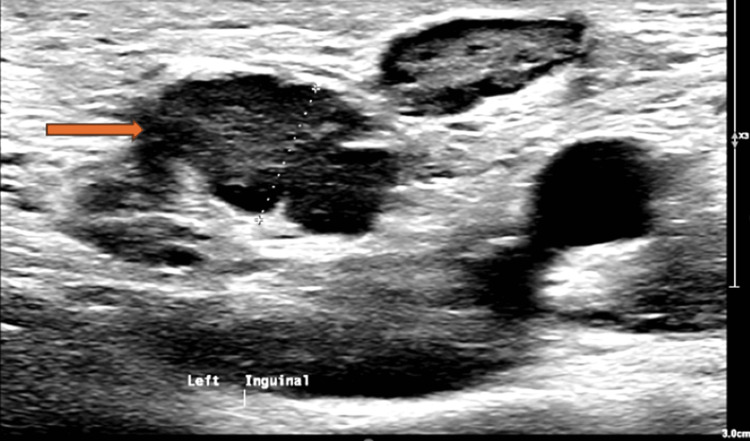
Left inguinal lymphadenopathy on ultrasound
